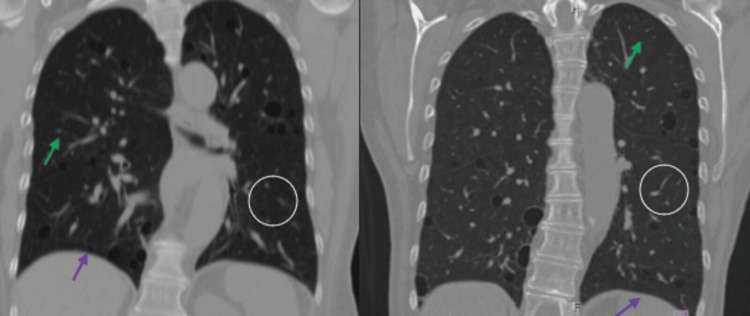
Chest CT scan performed initially in 2022 and during follow-up in 2024: pulmonary window with coronal reconstruction. In two years, there is no evidence of thickening of the interlobular septa (green arrow), areas of consolidation, nodules (white circle), or pleural effusion (purple arrow).
